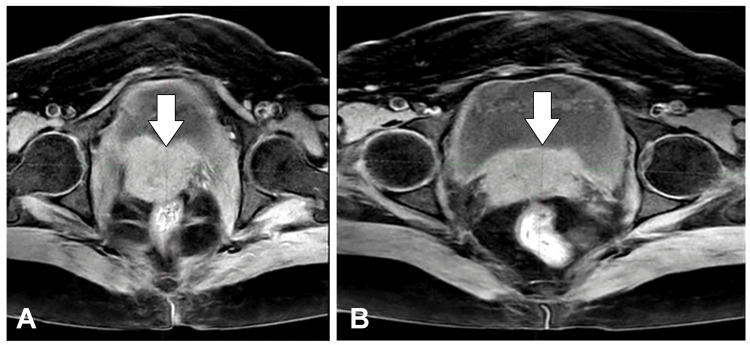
(A,B) Images of the T2-weighted MRI showing the recurrent disease in the vault of the patient (white arrows) MRI: magnetic resonance imaging
